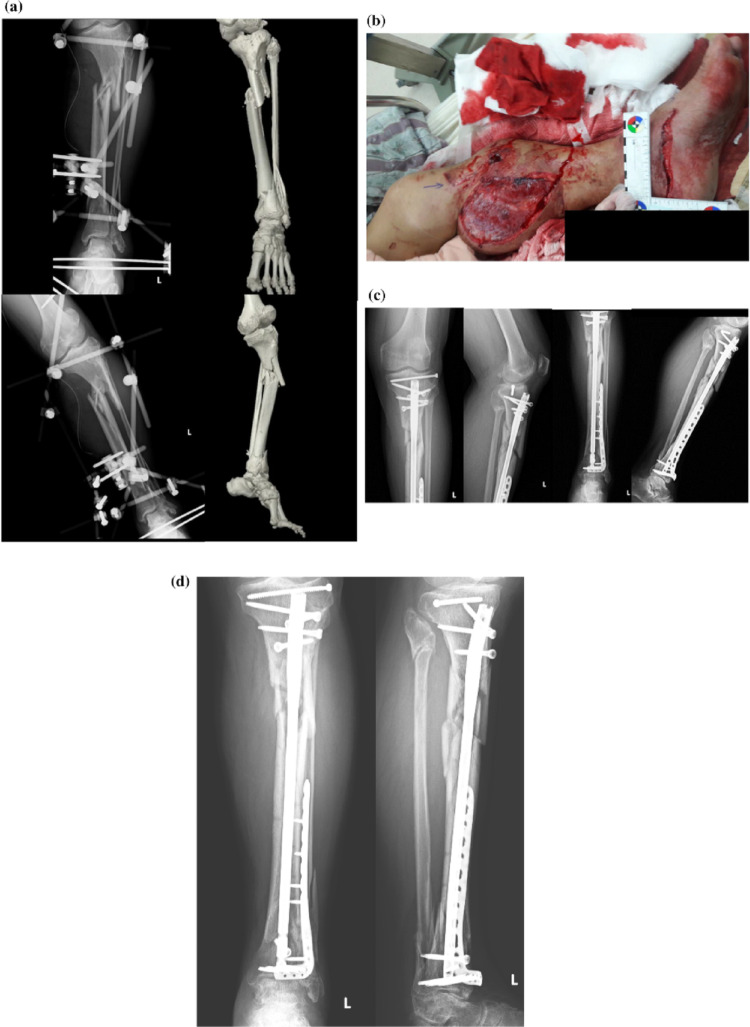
Representative case of a 57-year-old man after his left lower limb was hit by a steel rod during work. a Radiographs and CT of a left tibia AO/OTA 42B3 + 43C2.3 fracture. b Photograph showing a Gustilo open type III fracture with severe soft tissue damage. c Post-operative radiographs after the patient was operated through a nail-plate construct. d Radiograph 6 months after the operation showing bone union. CT: computed tomography, AO/OTA: Arbeitsgemeinschaft für Osteosynthesefragen/Orthopedic Trauma Association
